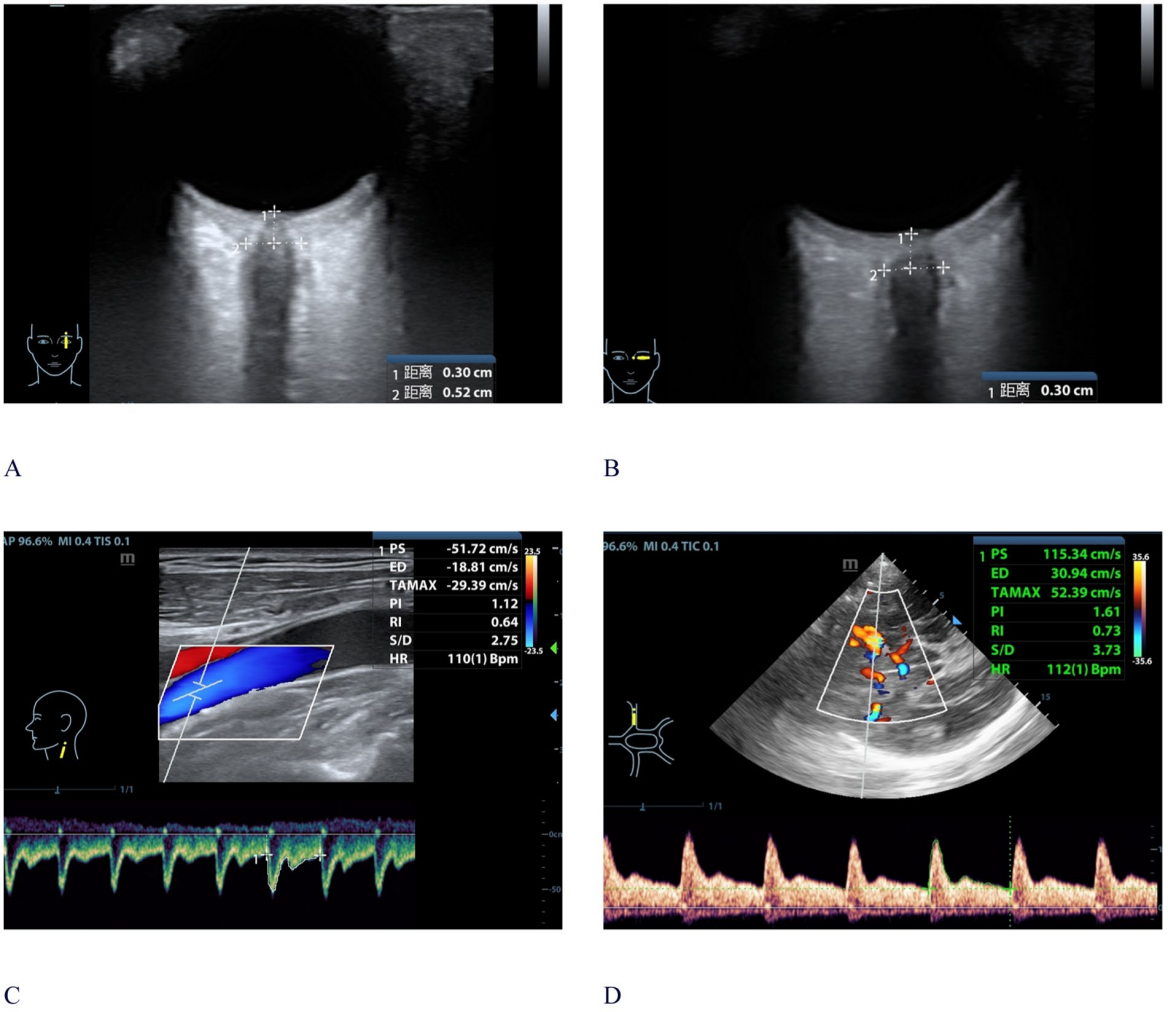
Bedside ultrasonographic measurements of CCAU and ONSD. (A) ONSD ultrasonography in the sagittal plane; (B) ONSD ultrasonography in the transverse plane; (C) CCAU measurement of ICA; (D) CCAU measurement of MCA. A 59-year-old male patient with a 24 h averaged ICPi of 23.6 mmHg, monitored following DC. CCAU and ONSD measurement were conducted within 24 h after ICPi. The ONSD value was 5.2 mm, with ICAPI: 1.12 and MCAPI: 1.61. The PI-ratio was greater than 1. CCAU, Cervical-Cerebral Arterial Ultrasound; ONSD, optic nerve sheath diameter; DC, decompressive craniotomy; ICPi_,_ invasive intracranial pressure; PI, pulsatility index; MCAPI_,_ pulsatility index of middle cerebral artery; ICAPI_,_ pulsatility index of internal carotid artery; PI-ratio, MCAPI/ICAPI; ICA, internal carotid artery; MCA, middle cerebral artery.
